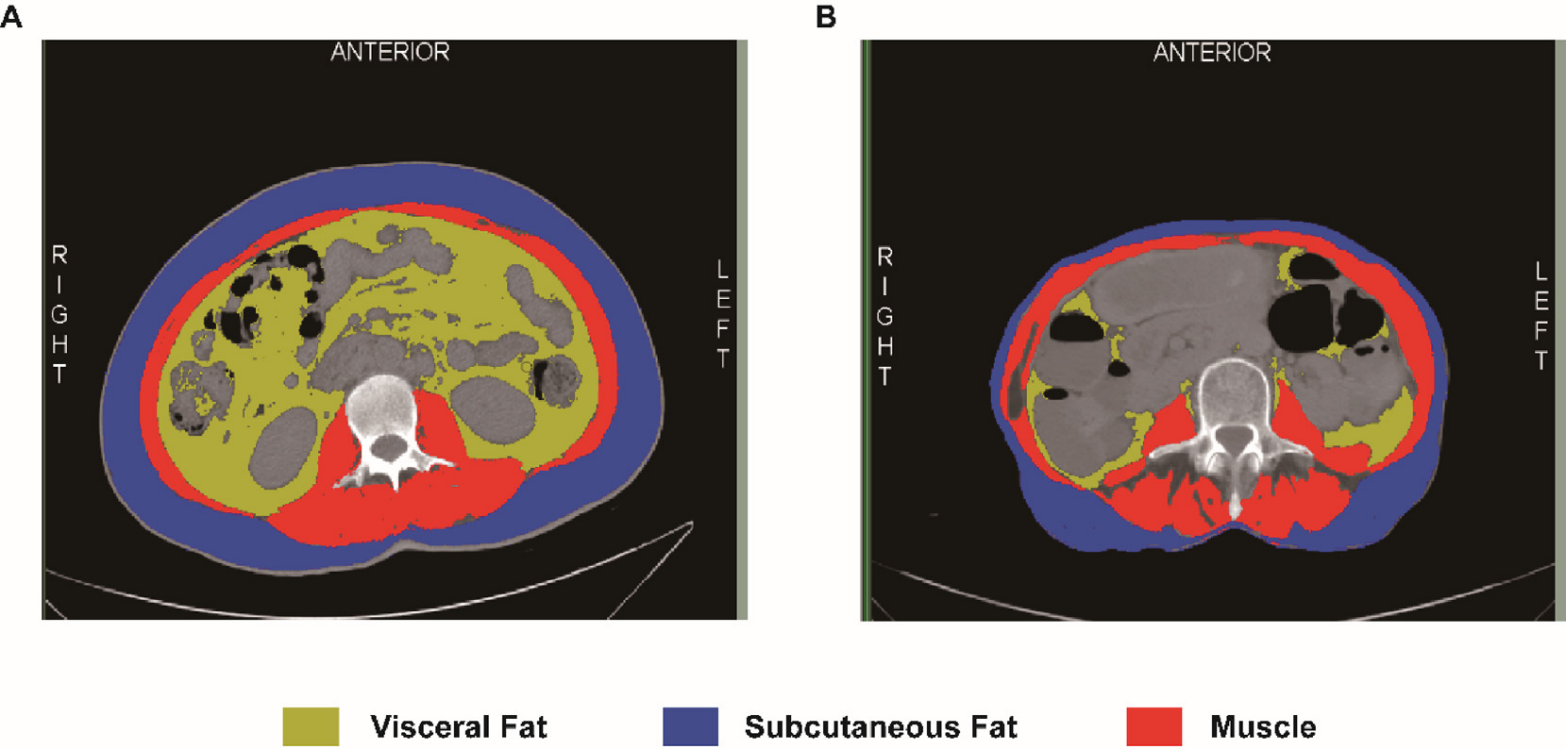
Body composition measurement of L3 horizontal abdominal CT cross-section. (A and B) Cross-sectional CT images were taken at the level of the third lumbar vertebra. The yellow area represents visceral fat, with an HU threshold of −150 to −50 HU; The blue area represents subcutaneous fat, with an HU threshold of −190 to −30 HU; The red area represents total skeletal muscle, with an HU threshold of −29 to 150 HU. CT: Computed tomography; HU: Huntsfield unit.
